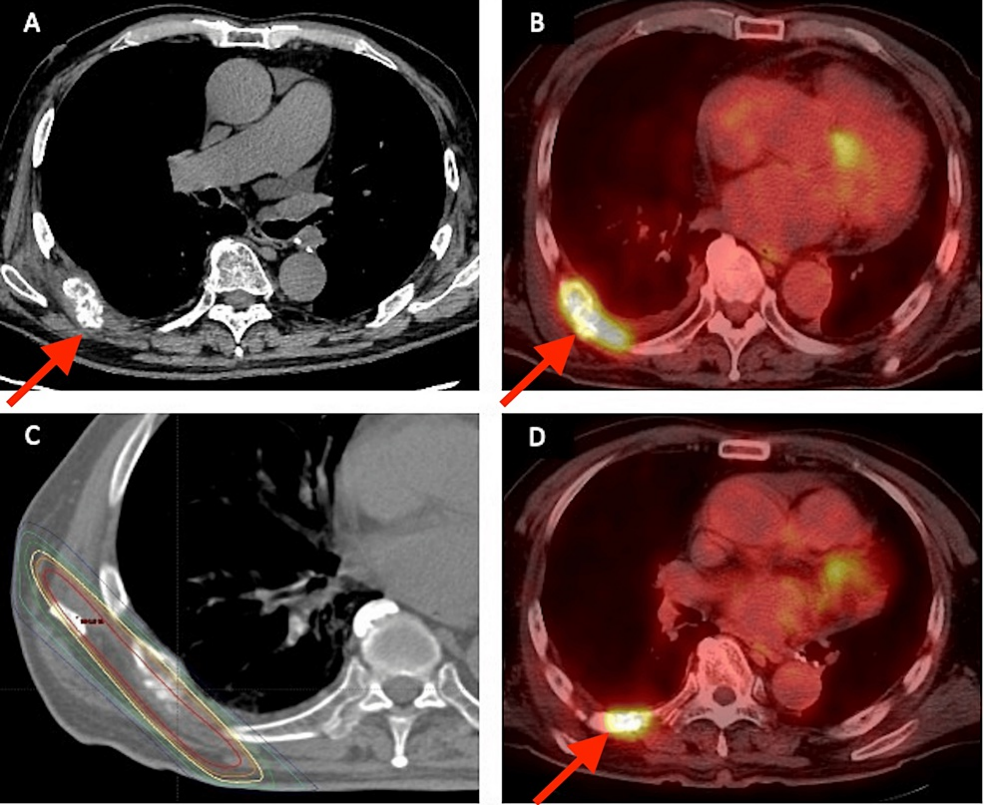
Imaging findings of right seventh rib metastasis and radiation therapy area (A) Chest CT showing a lytic lesion in the right seventh rib. (B) FDG-PET/CT showing abnormal FDG accumulation in the right seventh rib. (C) Radiation therapy (30 Gy in six fractions) for the right seventh rib metastasis. (D) FDG-PET/CT showing the recurrence at the edge of the irradiated field. CT, computed tomography; PET, positron emission tomography; FDG, fluorodeoxyglucose
